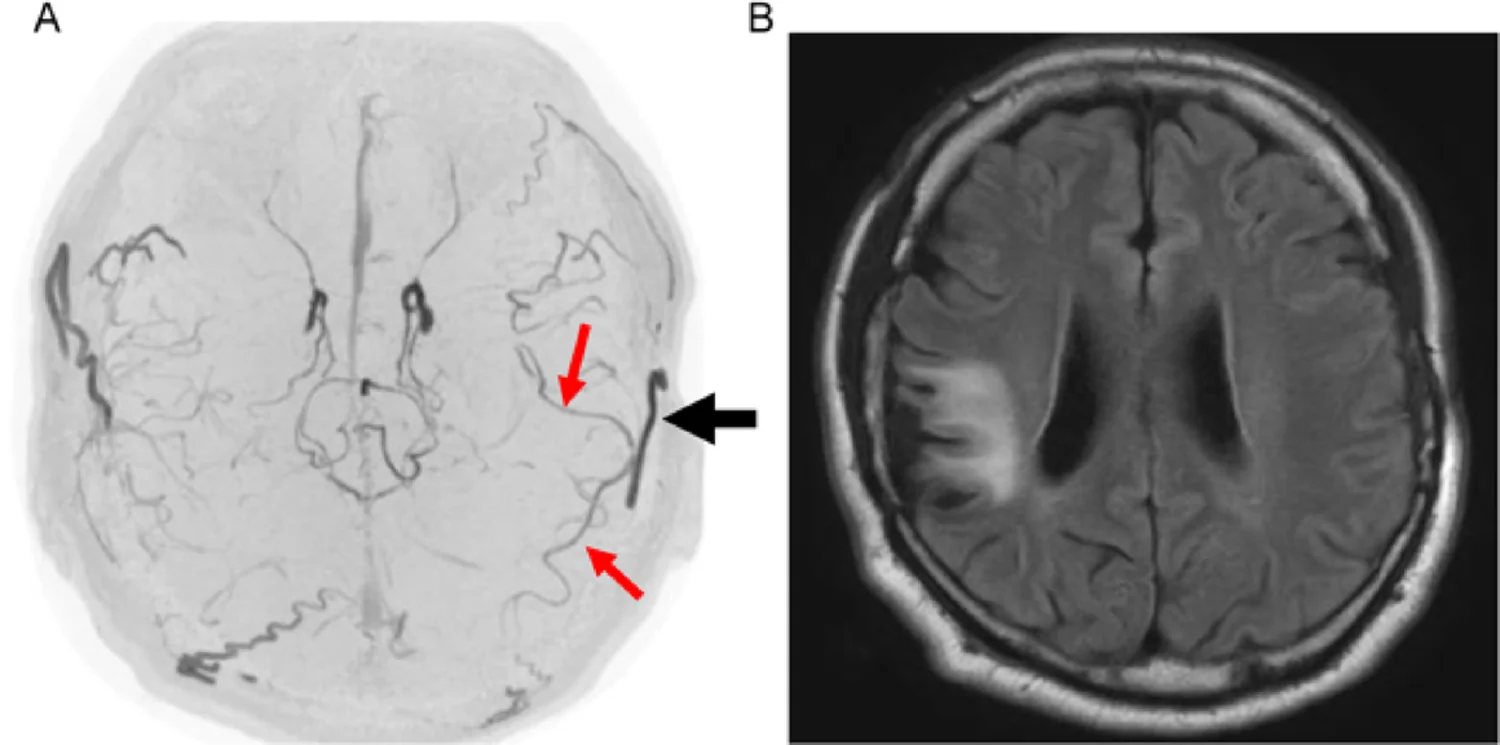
Case 2: Postoperative follow-up imaging demonstrating hemodynamic stabilization after revascularization and resolution of the FLAIR ivy sign (Phase 4). (A) Axial maximum intensity projection (MIP) MR angiography obtained five months after bypass surgery demonstrates good patency of the bypass graft (black arrow) with extracranial-to-intracranial collateral flow supplying the left cerebral hemisphere (red arrows), consistent with functional compensation for previously compromised posterior cerebral artery–derived superficial collateral pathways. (B) Follow-up fluid-attenuated inversion recovery (FLAIR) imaging shows resolution of the previously observed ivy sign in the left hemisphere. In the absence of new ischemic lesions, this finding is consistent with stabilization of cerebral hemodynamics after revascularization.
